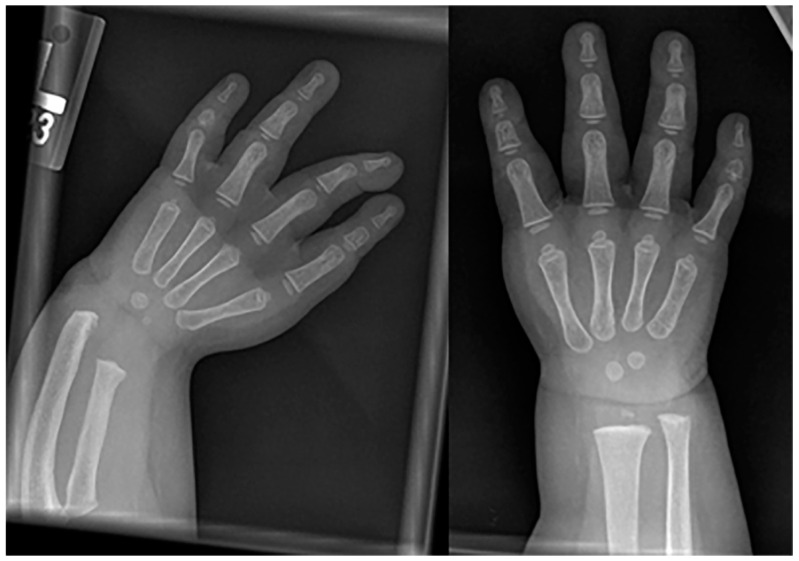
Frontal radiography of both forearms in a child with confirmed Fanconi anemia show left-dominant radial hypoplasia and bilateral aplasia of the thumbs.
